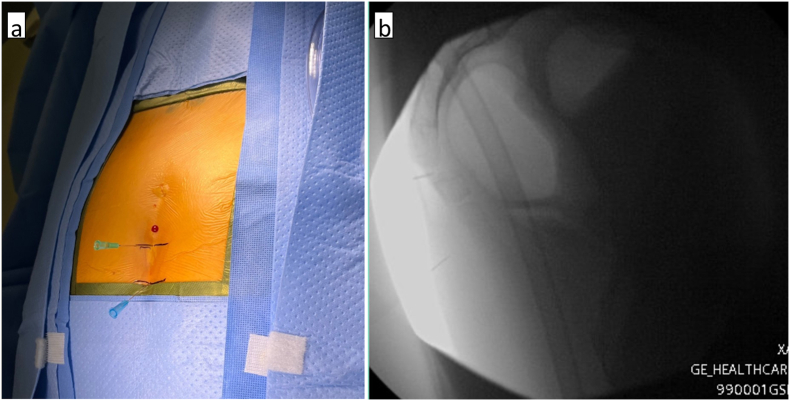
(a) Intraoperative localization with hypodermic needle. (b) Intraoperative localization with lateral fluoroscopic X-rays.
